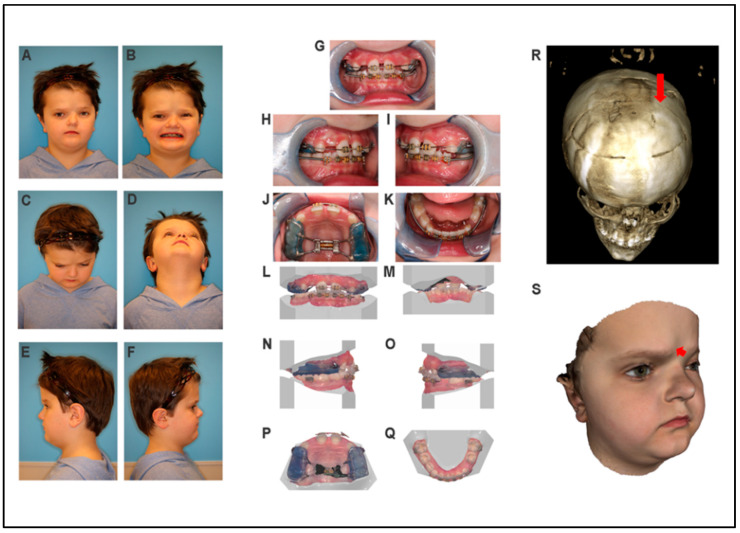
Clinical phenotype of a patient with Muenke syndrome (proband). (A–F) Two-dimensional photographs of the proband. (G–K) Intraoral photographs. (L–Q) Intraoral scan images. (R) Three-dimensional CBCT scan image. Red arrow shows the location of left craniosynostosis and repair. (S) Three-dimensional soft tissue image. Red arrow shows the frontal bossing.
